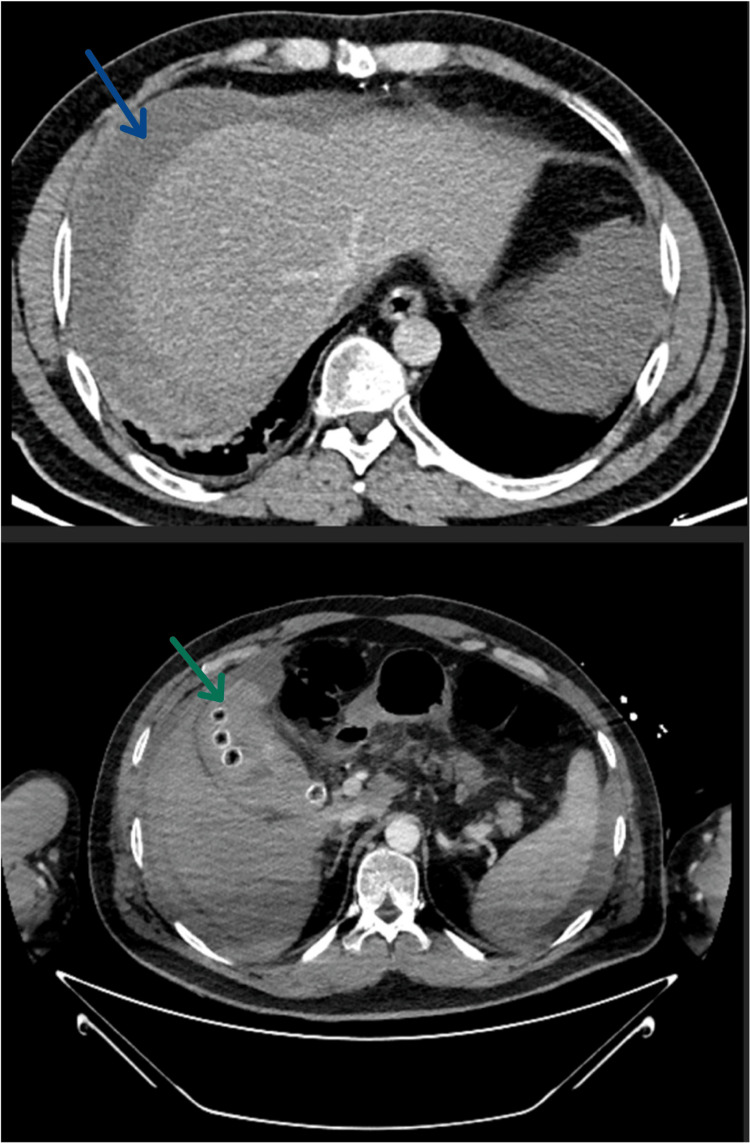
Abdominopelvic computed tomography Abdominopelvic computed tomography scan with angiography demonstrating an atypical gallbladder (green arrow) and a hemoperitoneum (blue arrow)
